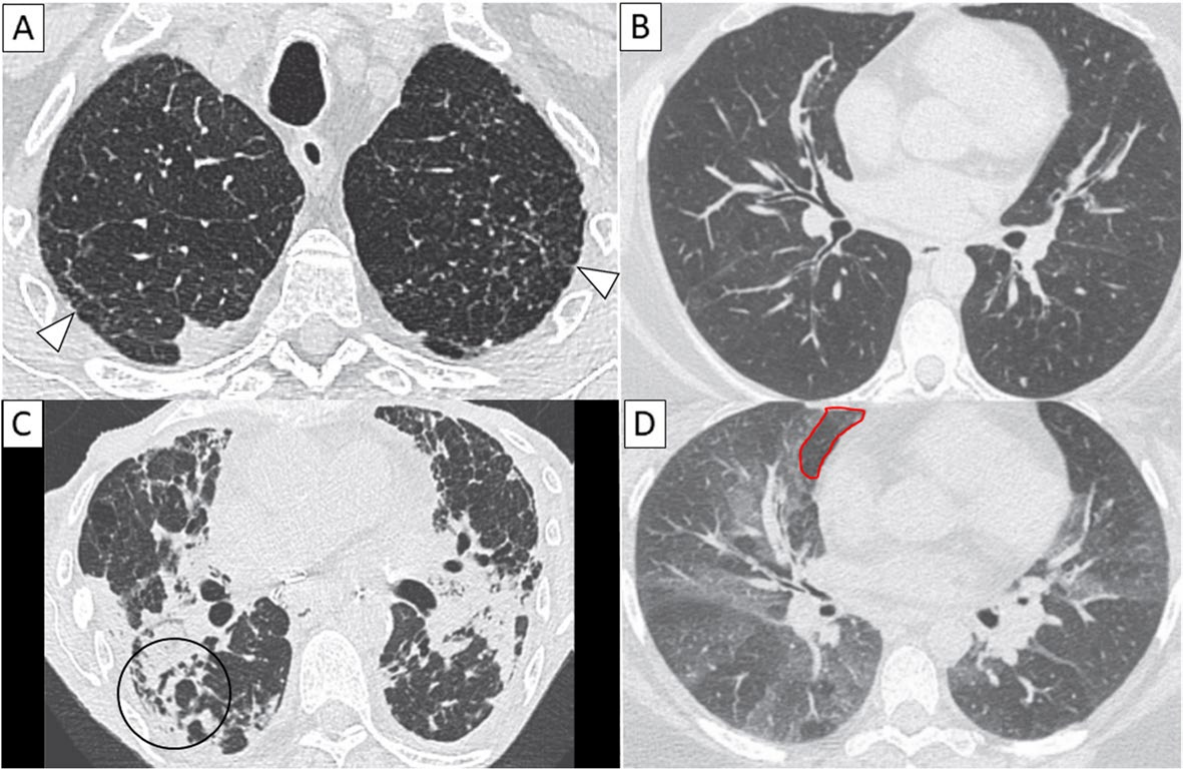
Unenhanced axial chest CT showing on the left side of the panel examples of RAS pattern (A, C), with subpleural reticular opacities (white arrowheads), volume loss, distortion, and traction bronchiectasis (black circle). On the right side of the panel, the images show examples of BOS pattern with a normal CT on deep inspiratory CT (B) and air trapping on the expiratory CT; an area is highlighted by a red circle (D)
